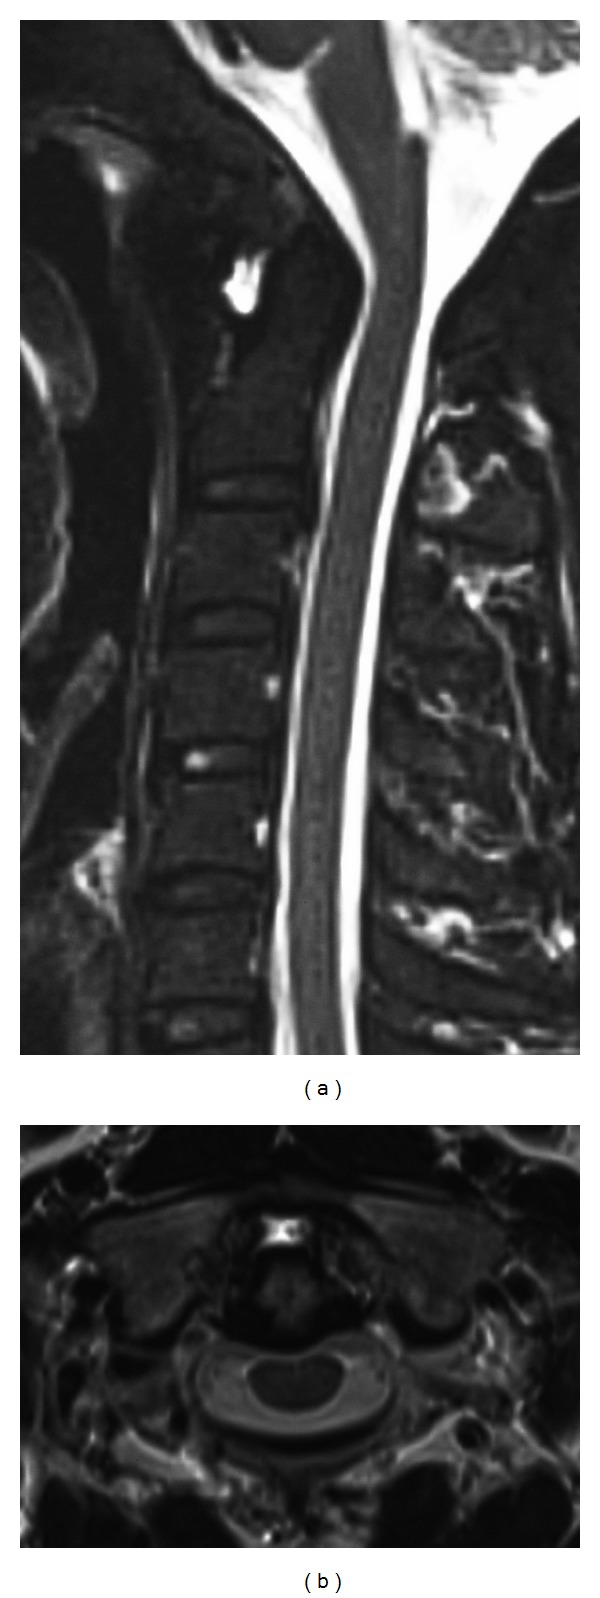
Cervical spine MRI. In (a) sagittal and (b) axial T2-weighted images, the high intensity area suggested fluid accumulation between the anterior arch of the atlas and odontoid process of the epistropheus.
